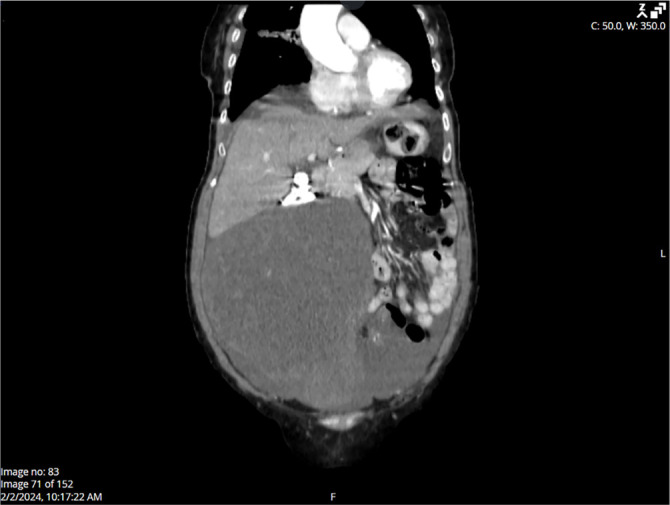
CT scan displaying a large oval solid lesion originating from the medial margin of the liver in the right hemiabdomen, extending toward the right ovarian fossa. The lesion exerts pressure on the surrounding vasculature and causes distortion of the right anterior abdominal wall.
